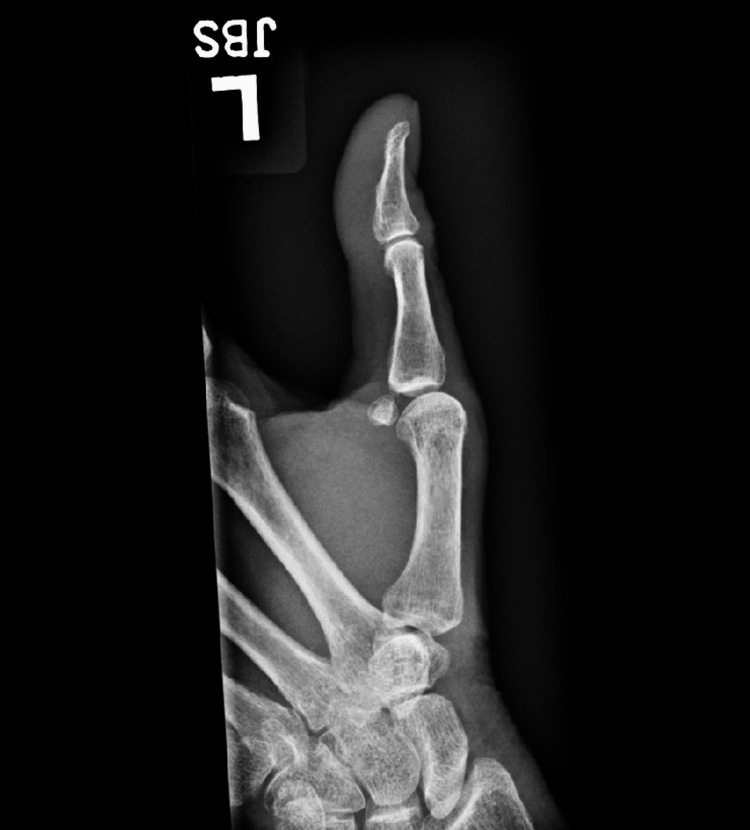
Post-reduction radiograph showing restoration of the normal positioning of the MCP joint and sesamoid bones. MCP: metacarpophalangeal.
